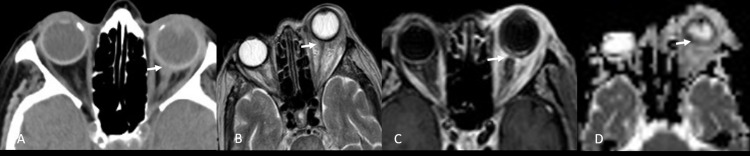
(A) Unenhanced axial computed tomography of the orbits demonstrates marked inflammatory changes involving the left globe and extending along the optic nerve. Proptosis and pre-septal edema. (B, C) Axial T2-weighted, postcontrast, fat-saturated image shows extensive enhancement of the periorbital soft tissue, detached choroid leaflet with generalized scleral thickening, irregular enhancing posterior choroid and optic nerve with enhanced chorio-retinal and scleral layers as well as intense ill-defined enhancement seen at the retro-scleral fat, along the optic nerve and ipsilateral extraocular muscles. (D) Diffusion-weighted and apparent diffusion coefficient (ADC) images showed restricted diffusion in the left-side subretinal fluid, compatible with an abscess collection "arrow", intraocular infection, and panophthalmitis.
